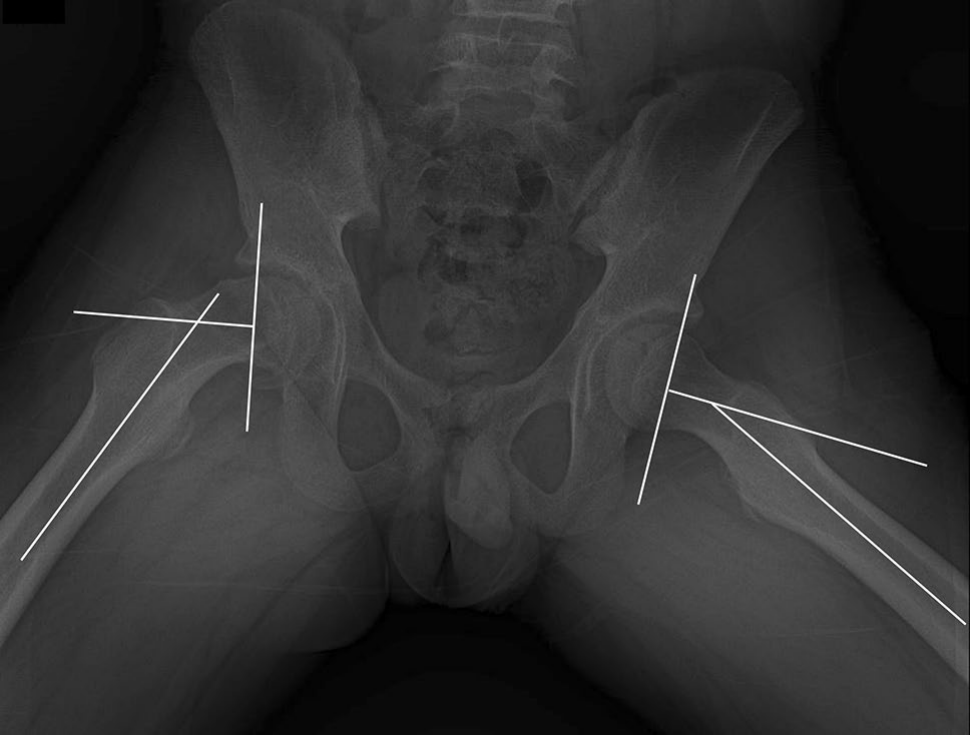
Measurement of the Southwick angle on a frog-leg lateral radiograph.
